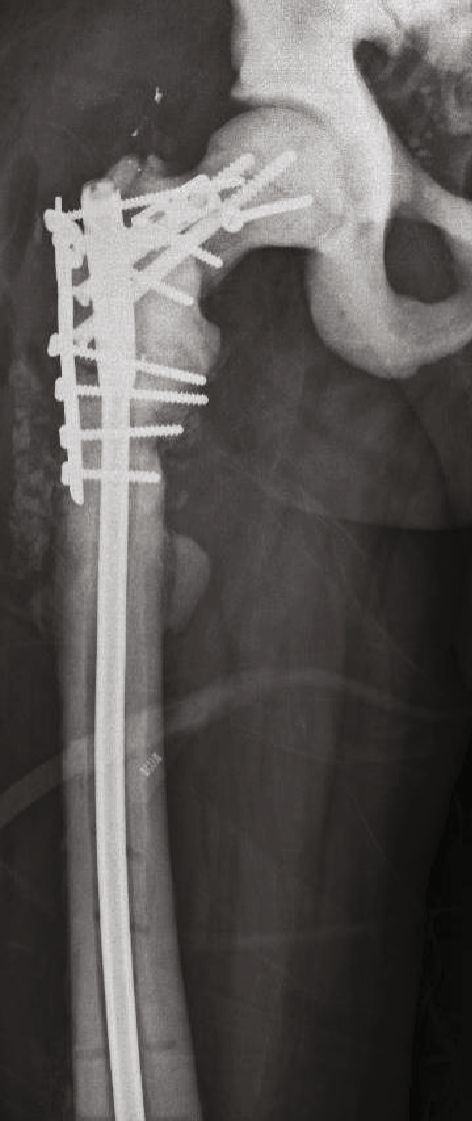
Postoperative anteroposterior radiograph of the femur after the last revision surgery.
